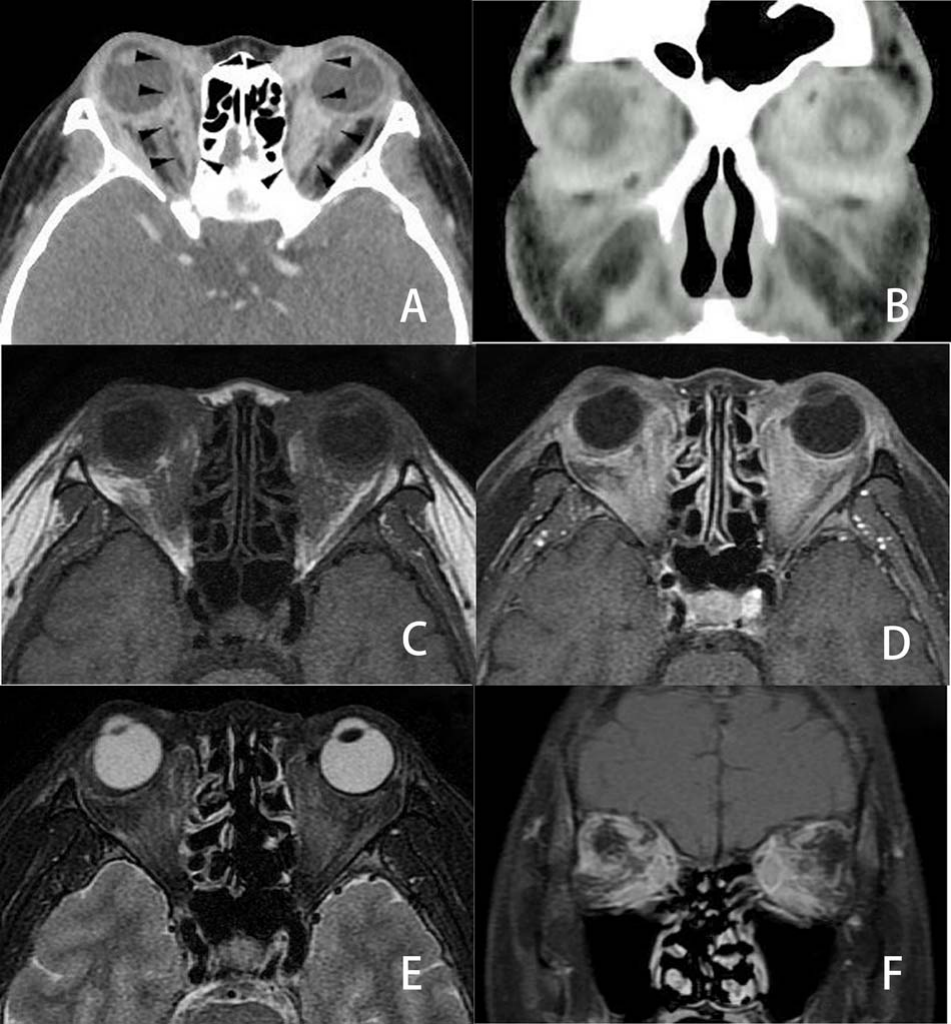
Three-dimensional imaging findings of orbital metastasis from breast cancer. Axial (A) and coronal (B) CT with contrast showed abnormal soft tissue enhancement (arrow) along the eyeball in the medial to retrobulbar portions of the bilateral orbits. The enhancement of the left orbit was greater than that of the right orbit. Axial MRI (C, D, E) also revealed an ill-defined tumor involving the soft tissues at the same portion where the soft tissue enhancement was seen on CT in the bilateral orbits. These orbital tumors were hypointense to fat tissue on axial T1-weighted imaging (C) and slightly enhanced on axial T1-weighted imaging with contrast (D) but slightly hyperintense to fat tissue on axial fat-suppressed T2-weighted imaging (E). Coronal fat-suppressed T1-weighted imaging with contrast (F) showed heterogenous irregular enhancement in the retrobulbar portion of the bilateral orbits. CT and MRI revealed no destruction of the eyeball, optic nerve or orbital bony wall.
